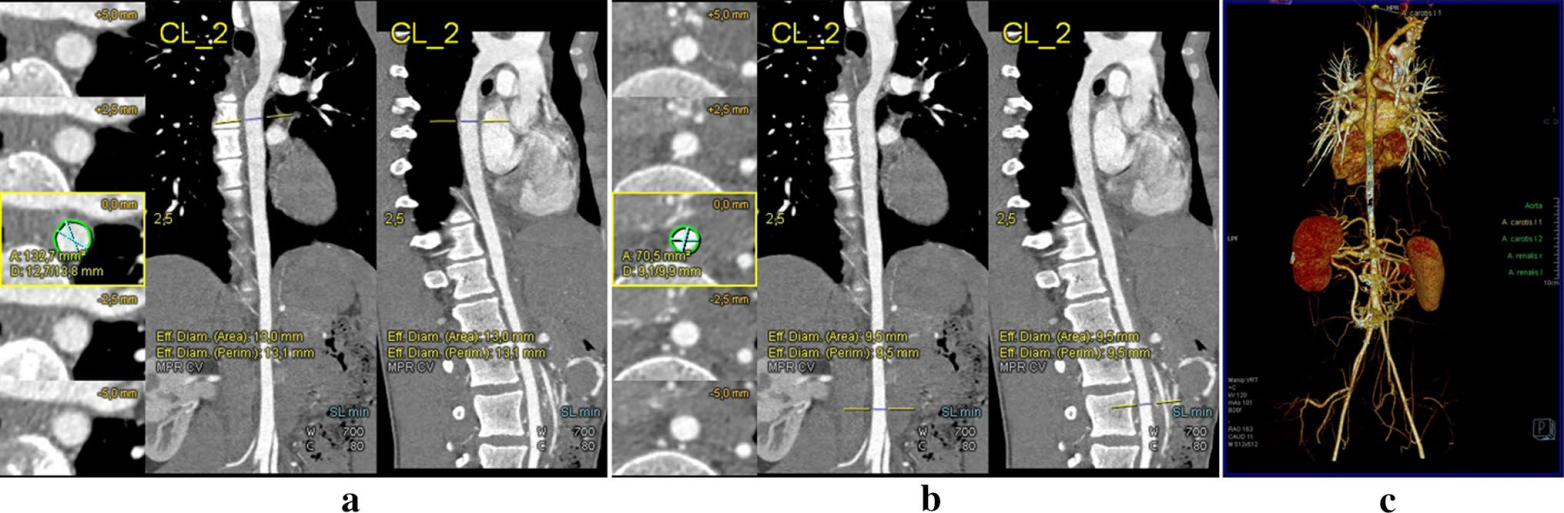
a, b, c Computed tomography angiography (CTA): long-segment coarctation of descending aorta beginning just after branching of the left subclavian artery. a, b Maximum intensity projection (MIP) reconstruction showing narrowing of the descending aorta at different levels. c Volume rendering technique (VRT)
